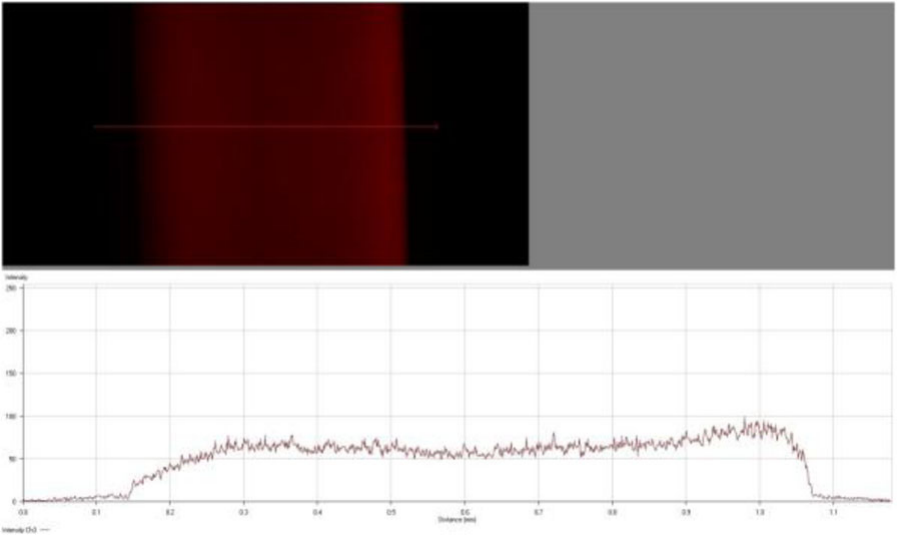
Control sample, no ultrasound applied. The X­direction is along the ultrasound beam, the Y­axis is the fluorescent intensity. The profile shows the cross­section the sandwich gel
